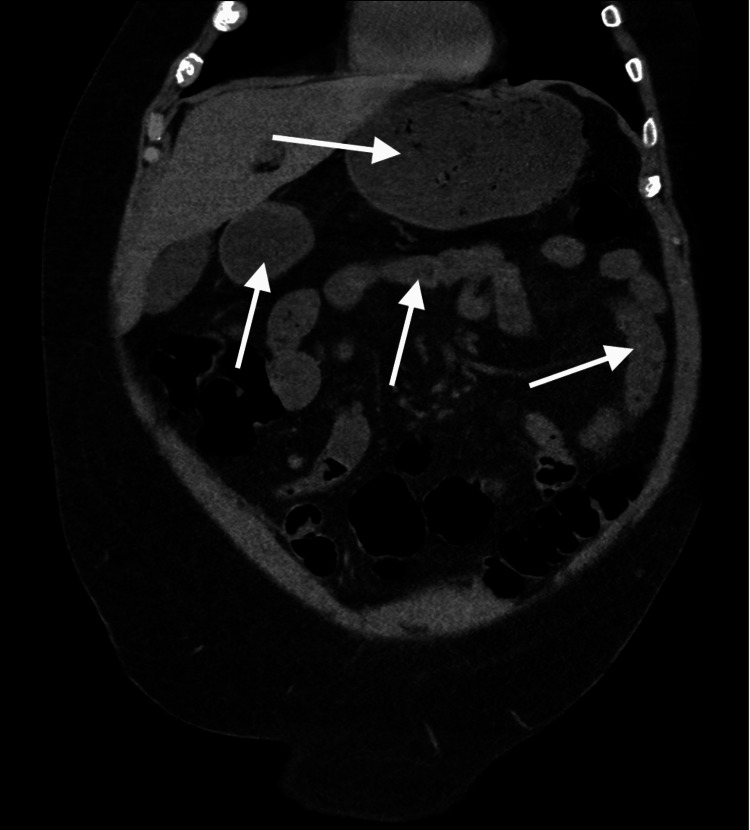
Computed tomography scan, coronal view, showing multiple loops of fluid-filled bowel (white arrows), consistent with the clinical picture of a diarrheal state, in this case presumed to be traveler’s acute gastroenteritis
